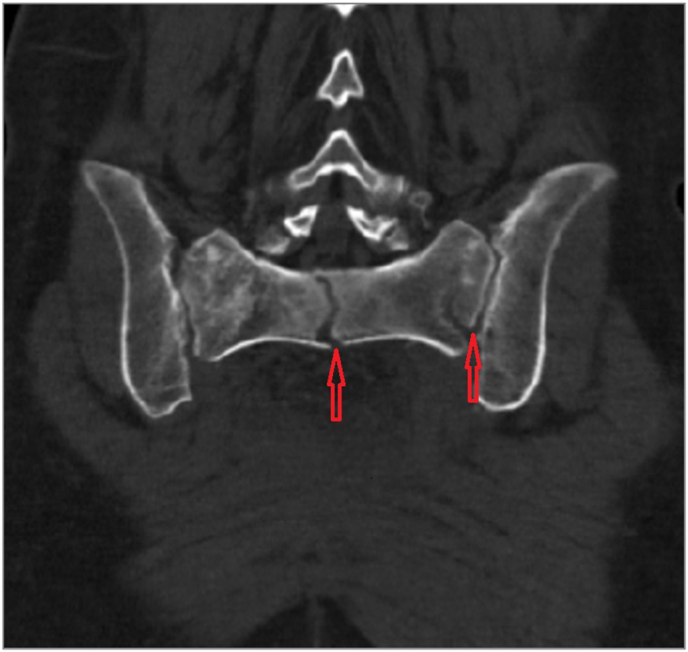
55 + 7 female patient 4 years after high level resection (S1-S2) for osteosarcoma with fractures (red arrows) of the residual part of the sacrum (Denis zones I + III), CT reconstruction in a coronal plane.
